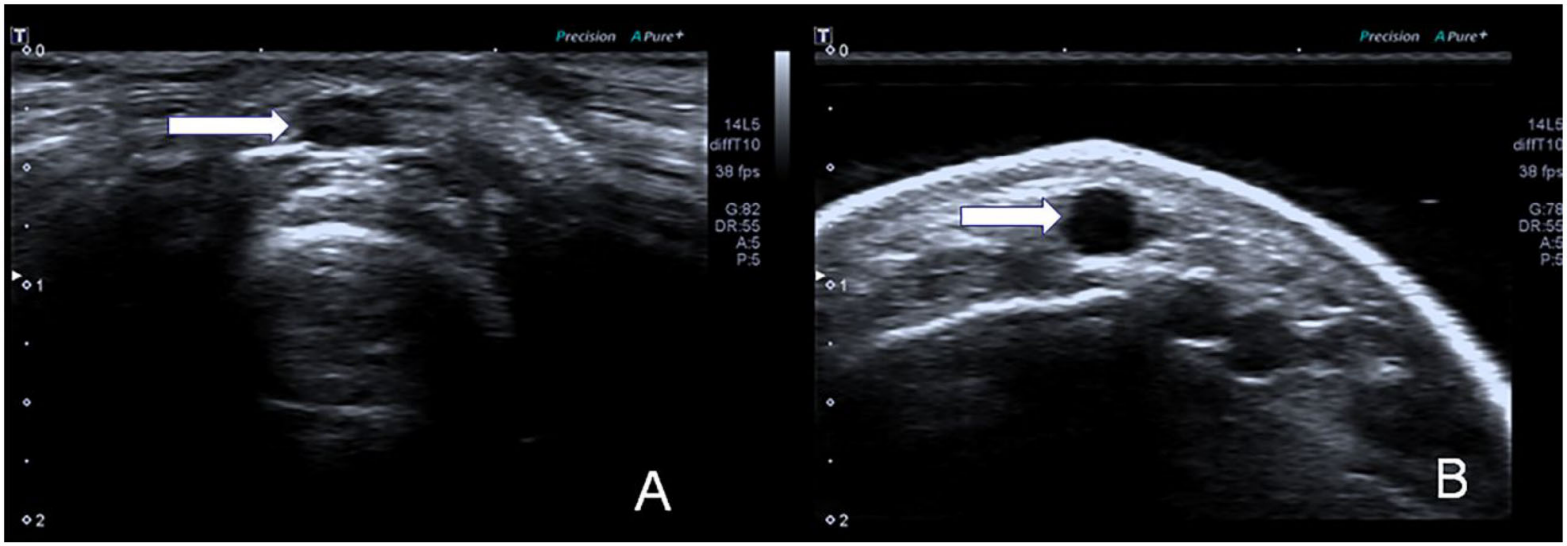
Ultrasound images of the cephalic vein (arrow) displayed *via* two approaches. (A) Contact ultrasonography. (B) Immersion ultrasonography.
